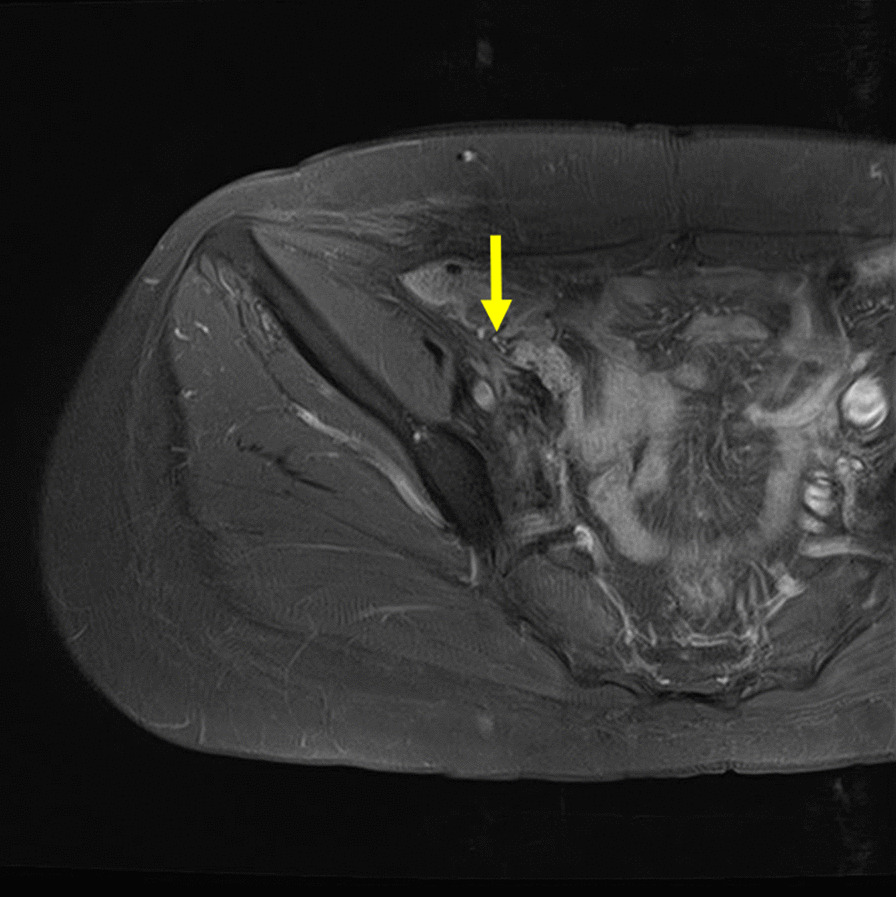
Post-gadolinium axial magnetic resonance imaging of pelvis. The lesion in the right pelvic wall shows no marked enhancement by intravenous administration of gadolinium (yellow arrow)
